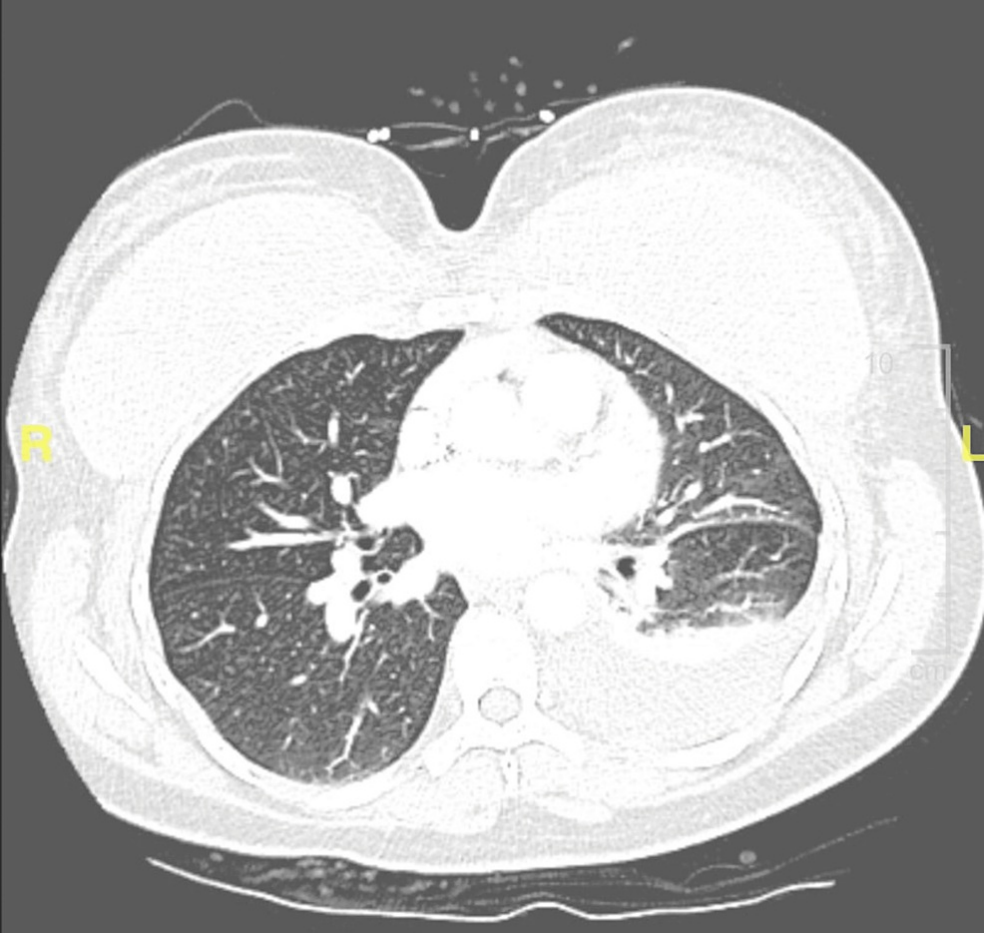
Computed tomography of the chest showing left pleural effusion
